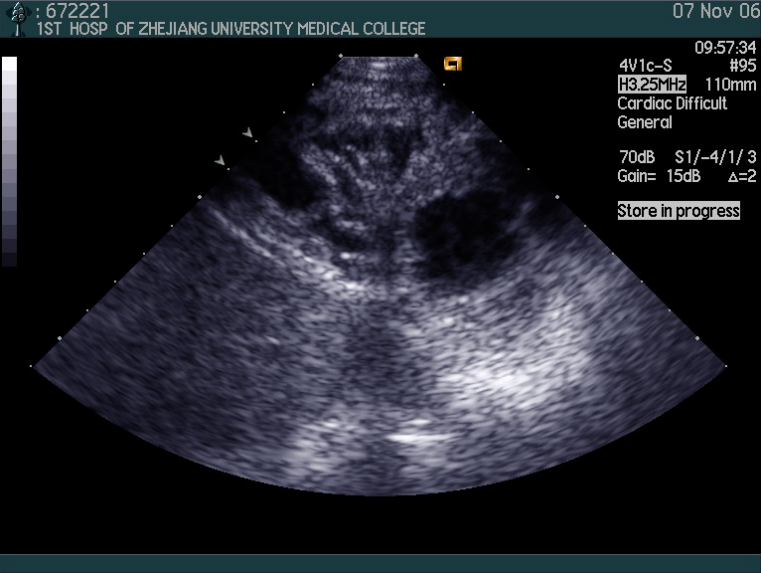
left ventricular short axis view at next to apex level showing the trabeculations of the right ventricular wall by two-dimension echocardiograms.
